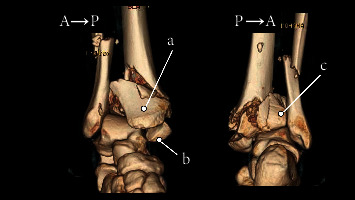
3D CT at the time of the injury. (a) Anterior fragments. (b) Medial fragments. (c) Posterolateral fragment.
